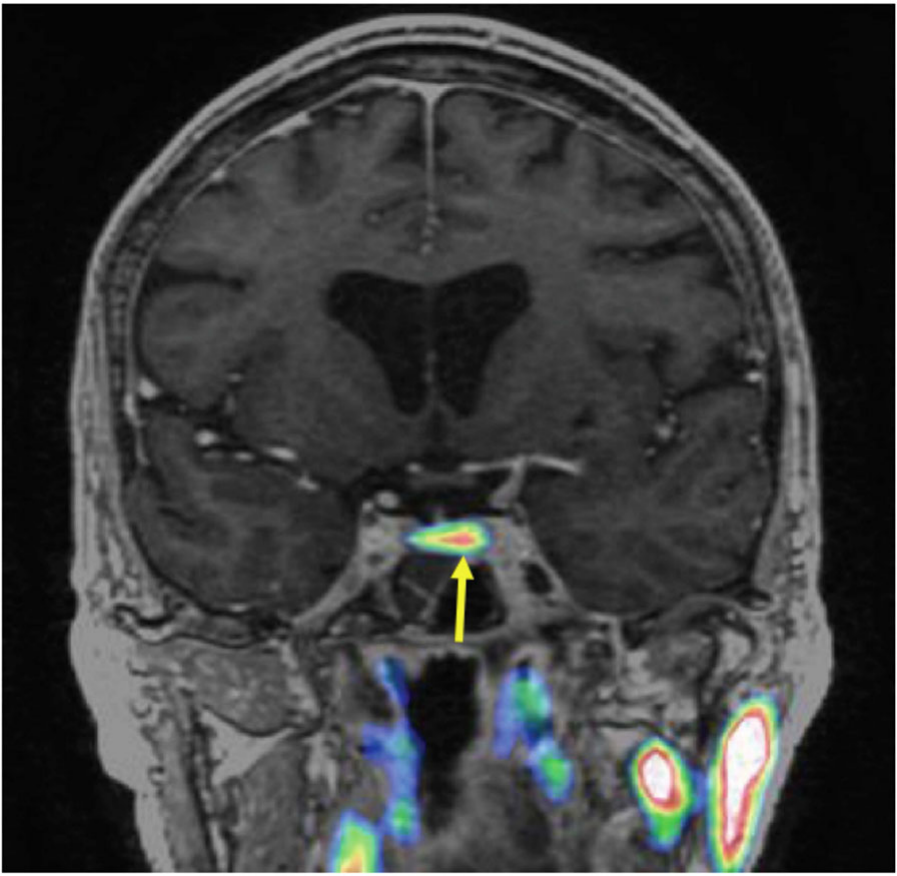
^11^C-methionine PET/CT coregistered with volumetric MRI. Coronal view
